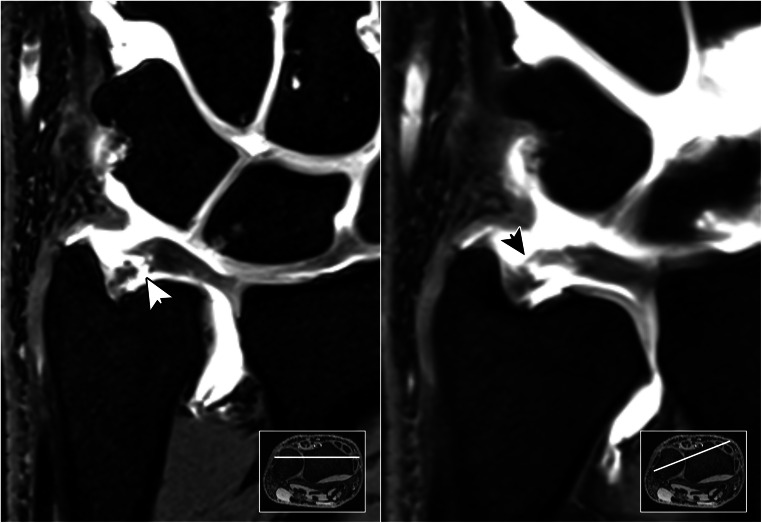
A 43-year-old man fell on his outstretched left hand. He noticed increasing pain on the ulnar side of the wrist. While no fracture was ascertained in radiography, orthogonal reformatting of MR arthrogram (left) suggested a complete tear in the ulnar portion of the TFCC (white arrow). Radial image reconstruction (right) revealed a high-grade partial tear with some dorsal fibers of the styloid lamina still intact (black arrow). Due to the confirmed discontinuity of the deep insertion, which functions as the main stabilizer of the distal radioulnar joint, refixation was recommended nonetheless
